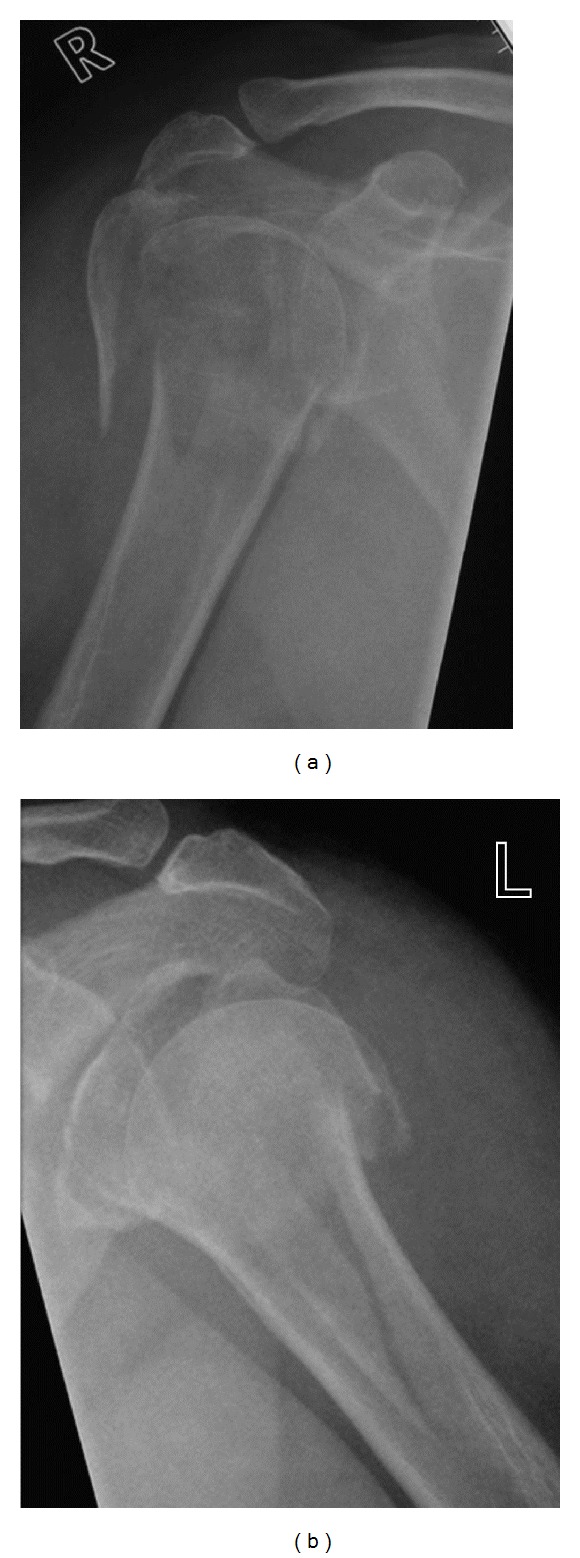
Preoperative radiographs demonstrating four-part proximal humeral fractures bilaterally.
